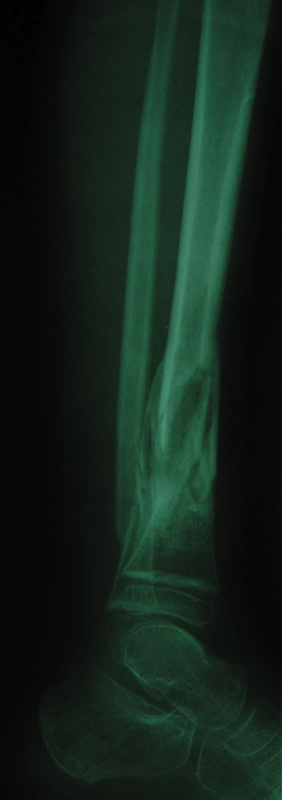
Lateral roentgenogram of the left leg 4 months postinjury demonstrates the fracture in appropriate alignment. The extruded fragment was incorporated into the callus of the fracture site.
